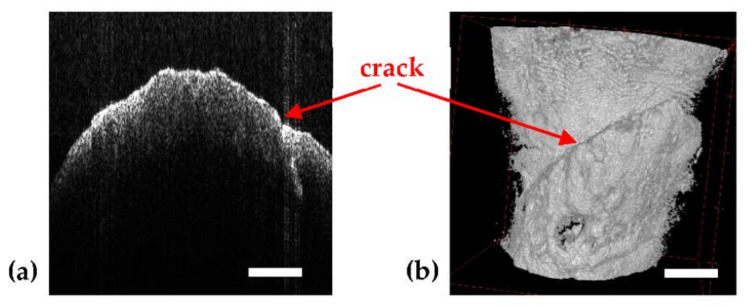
Example of a single OCT B-scan/optical cross section (a) and the corresponding 3D OCT image reconstruction (b), showing a crack in a tooth—example of OCT imaging showing the higher resolution capability compared to radiography, as approached in detail in the exploratory study in [28]. Scale: 1 mm.
